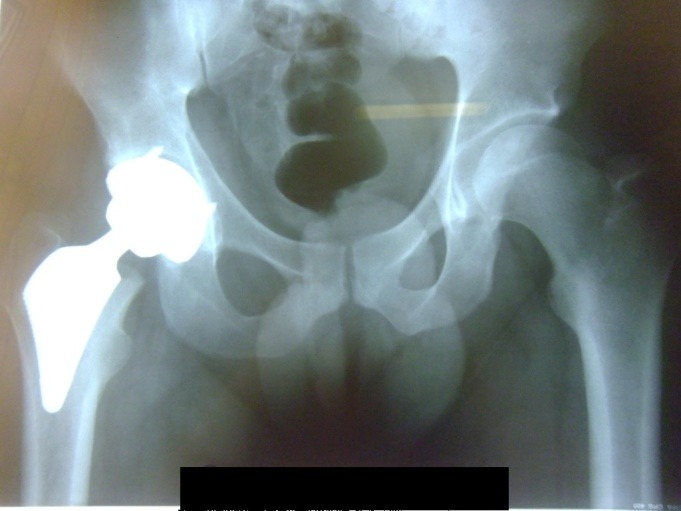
Postoperative X-Ray showing the implant
